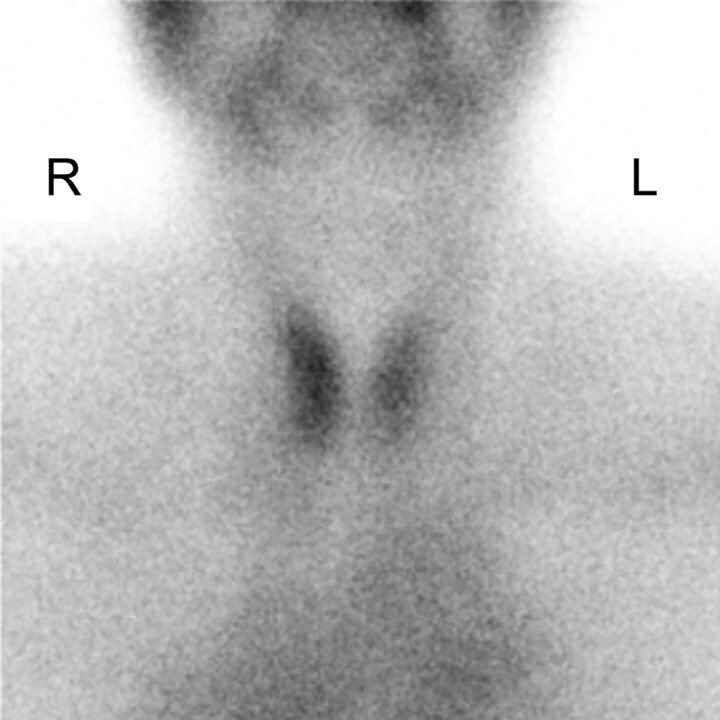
A ^99m^Tc-pertechnetate scintigram on admission shows normal uptake in the thyroid gland. L, left; R, right.
